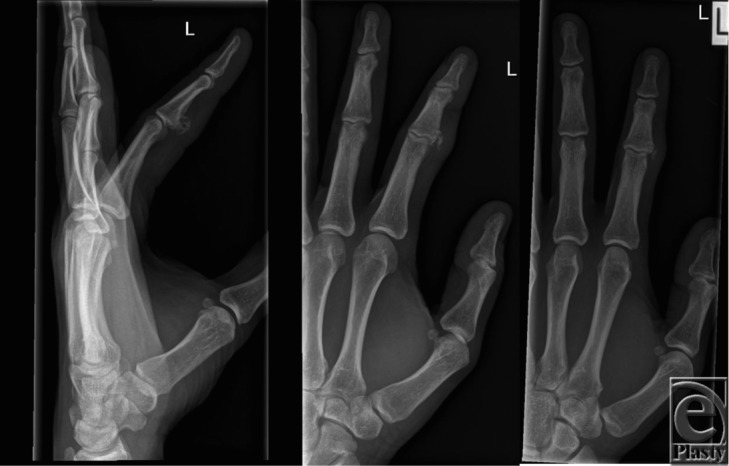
Radiograph at 6 months postoperatively, following initial exploration and removal. This shows a calcified mass at the previous operative site, separate from the underlying cortex.
